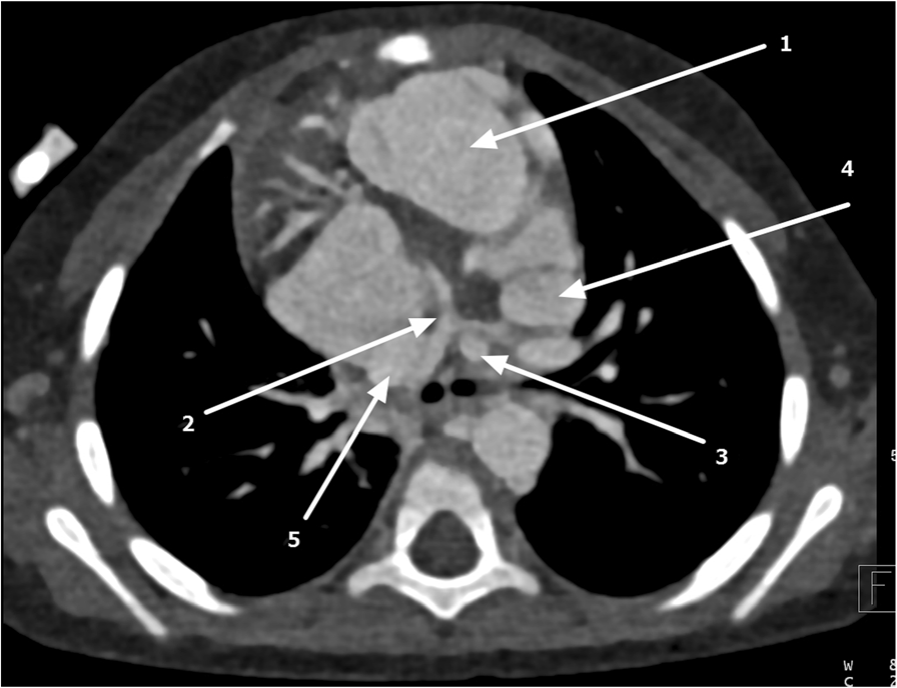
Axial cardiac computed tomography showing transposed ascending aorta 1 arising from morphologic right ventricle (1), hypoplastic main pulmonary artery (MPA) (2), left-sided patent ductus arteriosus (PDA) (3), and double-sided superior vena cava (4 and 5)
